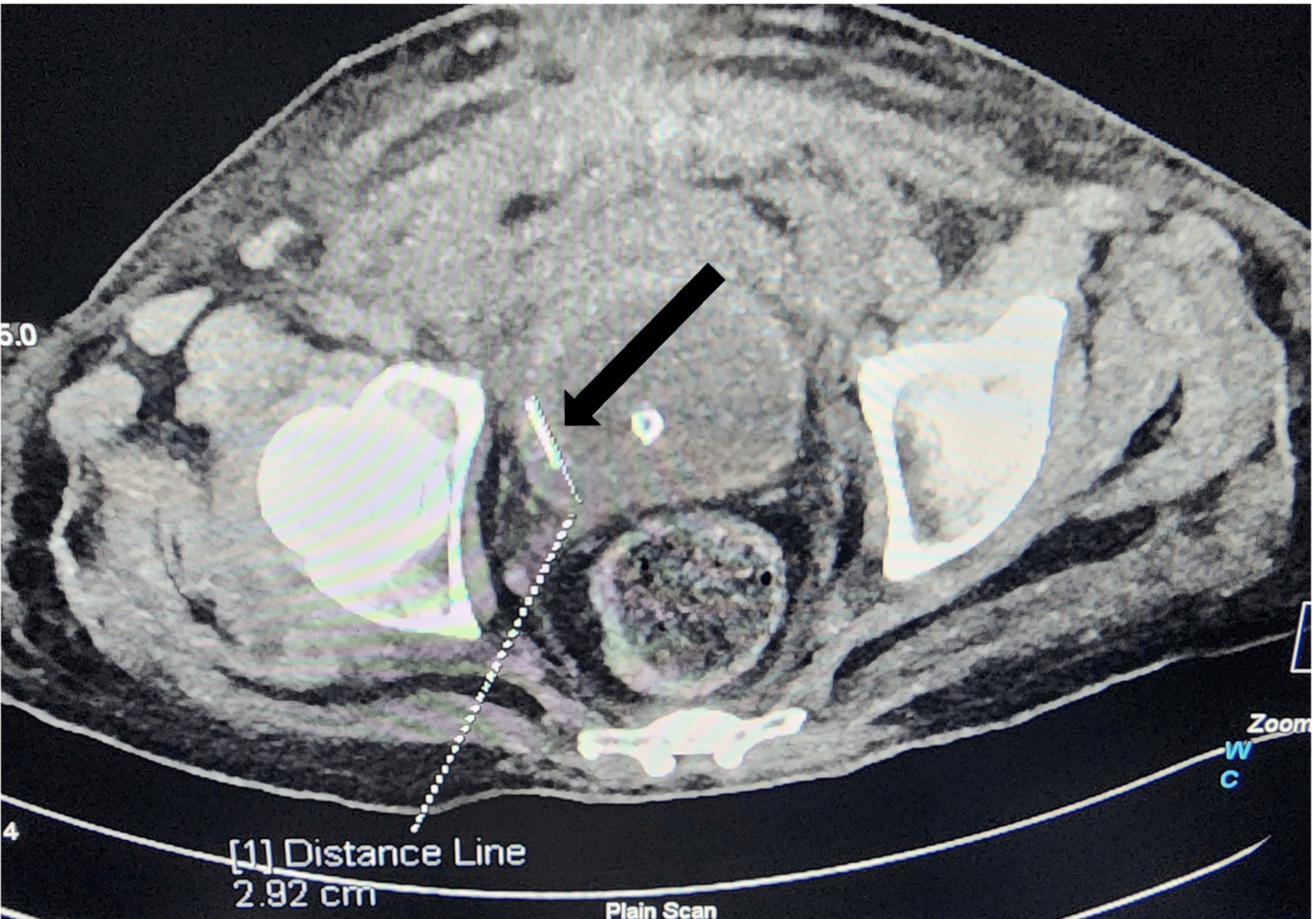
Computed tomography confirmation of an intravesical foreign body Axial non-contrast computed tomography scan of the pelvis demonstrating a linear radio-opaque foreign body within the urinary bladder lumen, adjacent to the right lateral wall (black arrow).
